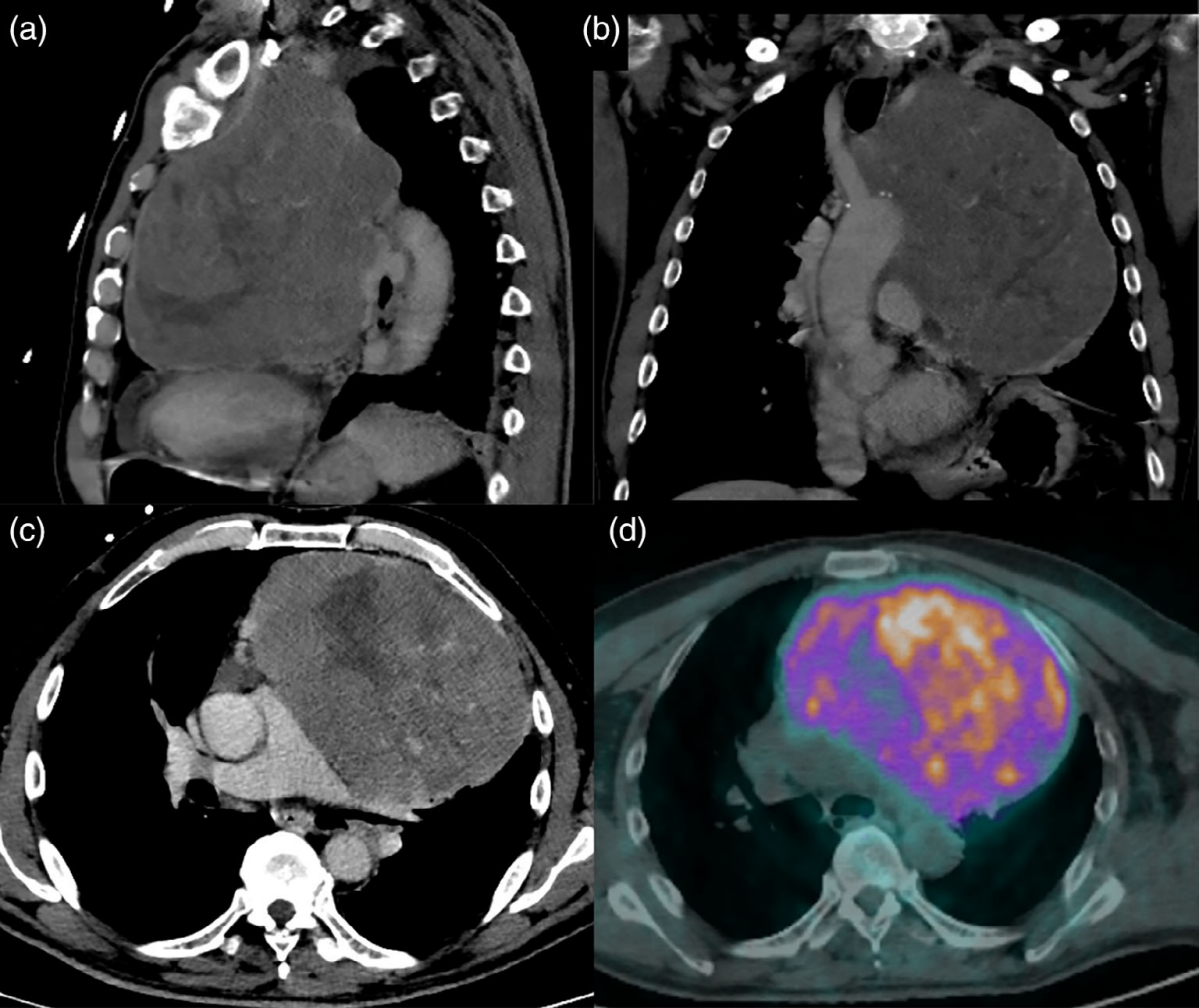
(a,b,c) Sagittal, coronal and axial contrast‐computed tomography (CT) showed the presence of a tumor mass measuring 16 × 12 × 18 cm localized in the anterosuperior mediastinum causing compression of the left cardiac chamber, aortic arch, origin of the supra‐aortic vessels, the common trunk of the pulmonary artery and the left main bronchus. (d) 68Ga‐DOTA‐TOC positron emission tomography (PET) showed high uptake limited to the mediastinal mass
